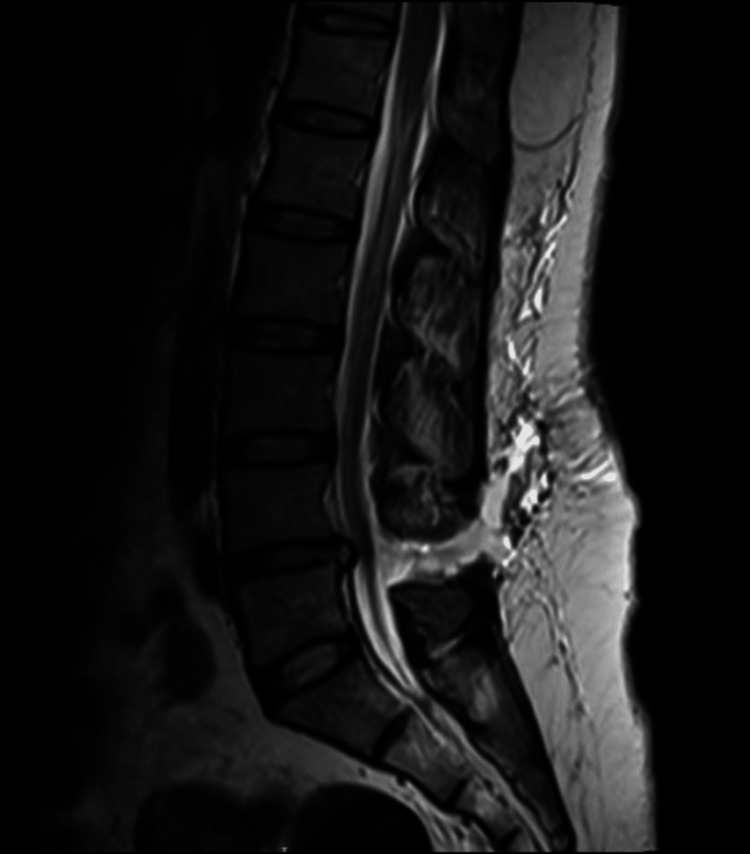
Lumbar spine MRI, sagittal T2, showing a large epidural fluid collection seen at the level of L4-L5 extending from the epidural space posteriorly through the posterior back muscles and to the superficial subcutaneous fat
